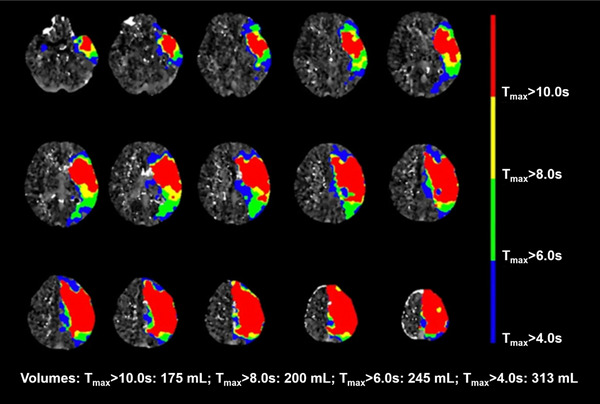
Imaging example of a fast stroke progressor CT perfusion scans of a patient with large volumes of tissue exhibiting high T_max_ values. The hypoperfusion intensity ratio in this patient is 0.71. This patient is very likely a fast stroke progressor who may benefit from cerebroprotective approaches during transfer times.
